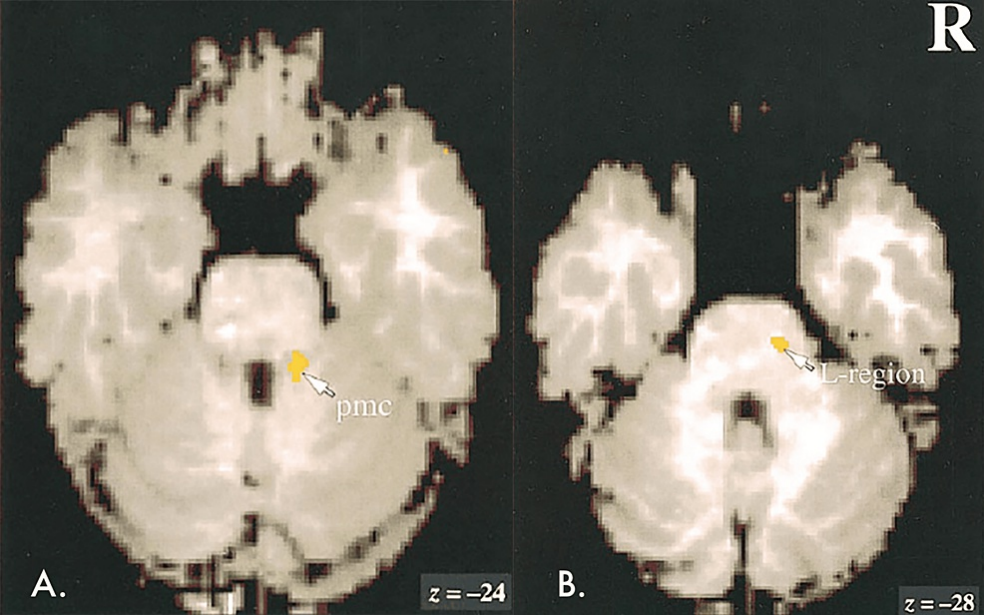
PET-CT of the brain, showing the pontine micturition center and L-region, located in the pons PMC: pontine micturition center; PET-CT: positron emission tomography-computed tomography Published with permission: Blok et al. (1997) [5]
